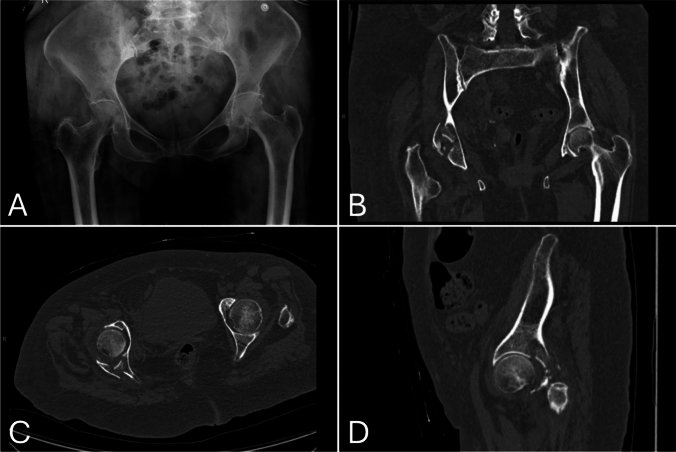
Anteroposterior (AP) radiograph of the pelvis A and coronal B, axial C, and sagittal D computed tomography (CT) images of the right hip obtained upon presentation to the emergency department following a ground-level fall. Imaging demonstrates a posterior column and posterior wall acetabular fracture
